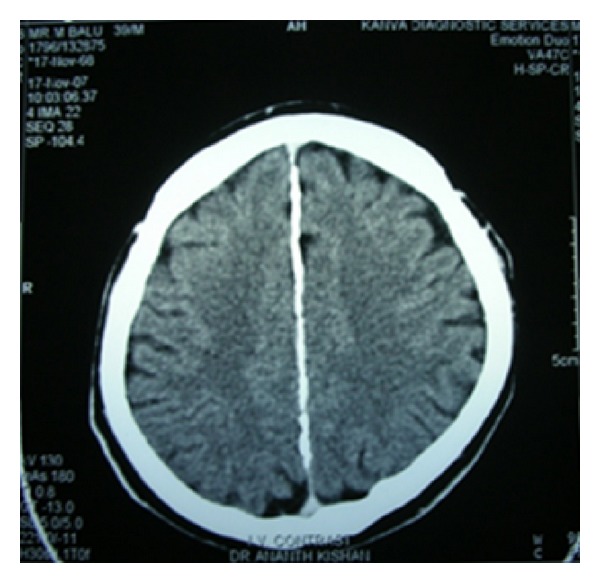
Axial CT of brain showing calcification of falx cerebri.
